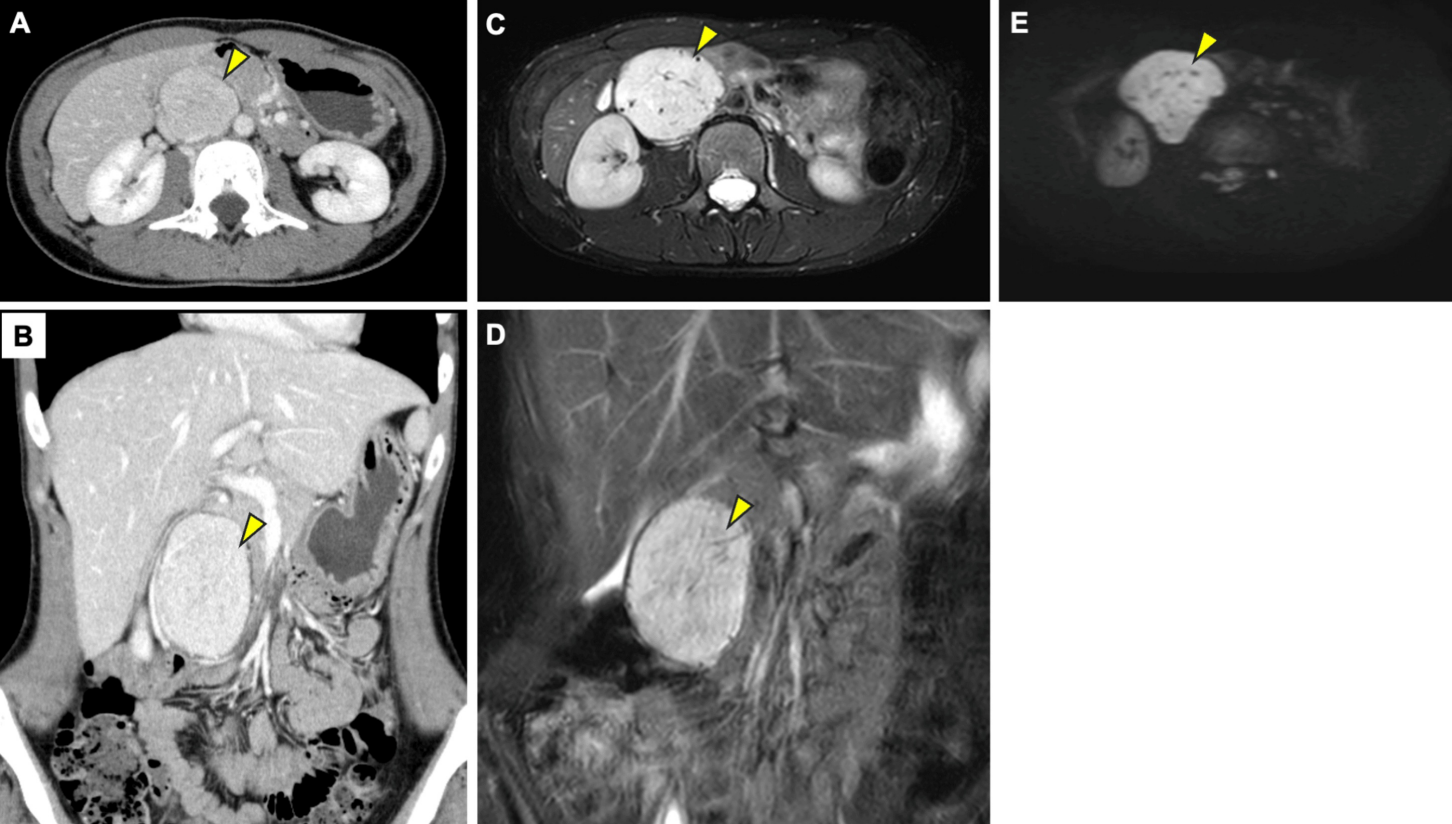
Abdominal imaging of the patient (A, B) Contrast-enhanced CT in the arterial phase demonstrates a well-defined mass (arrowheads) situated between the abdominal aorta and inferior vena cava, with intense enhancement. (C, D) T2-weighted MR images (axial and coronal) show the retroperitoneal tumor (arrowheads) as uniformly hyperintense with internal flow-voids suggestive of dilated vessels, with internal dilated vessel-like flow voids. (E) Diffusion-weighted MR image confirms high signal intensity within the lesion (an arrowhead).
